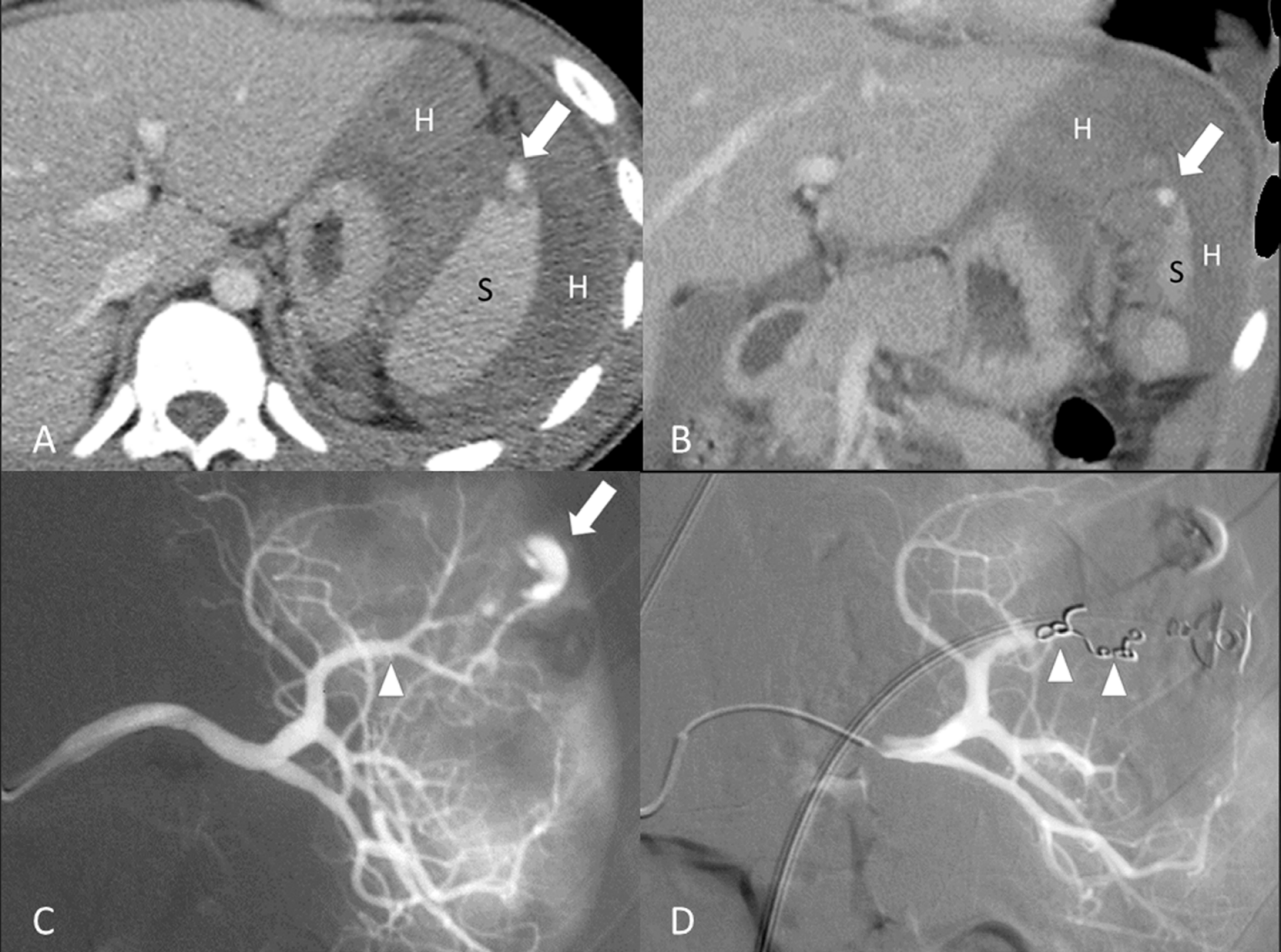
18-year-old male of grade II splenic injury underwent distal transcatheter arterial embolization (A, B) Contrast-enhanced axial and coronal CT at portal venous phase shows a pseudoaneurysm (arrow) at the lacerated spleen (S) surrounded by hemoperitoneum (H). (C) Selective angiography confirms the CT finding of a pseudoaneurysm (arrow) and identifies the feeding branch artery (arrowhead). (D) Angiography after a successful distal embolization shows deployment of metallic coils (arrowheads) at the branch artery and obliteration of pseudoaneurysm.
